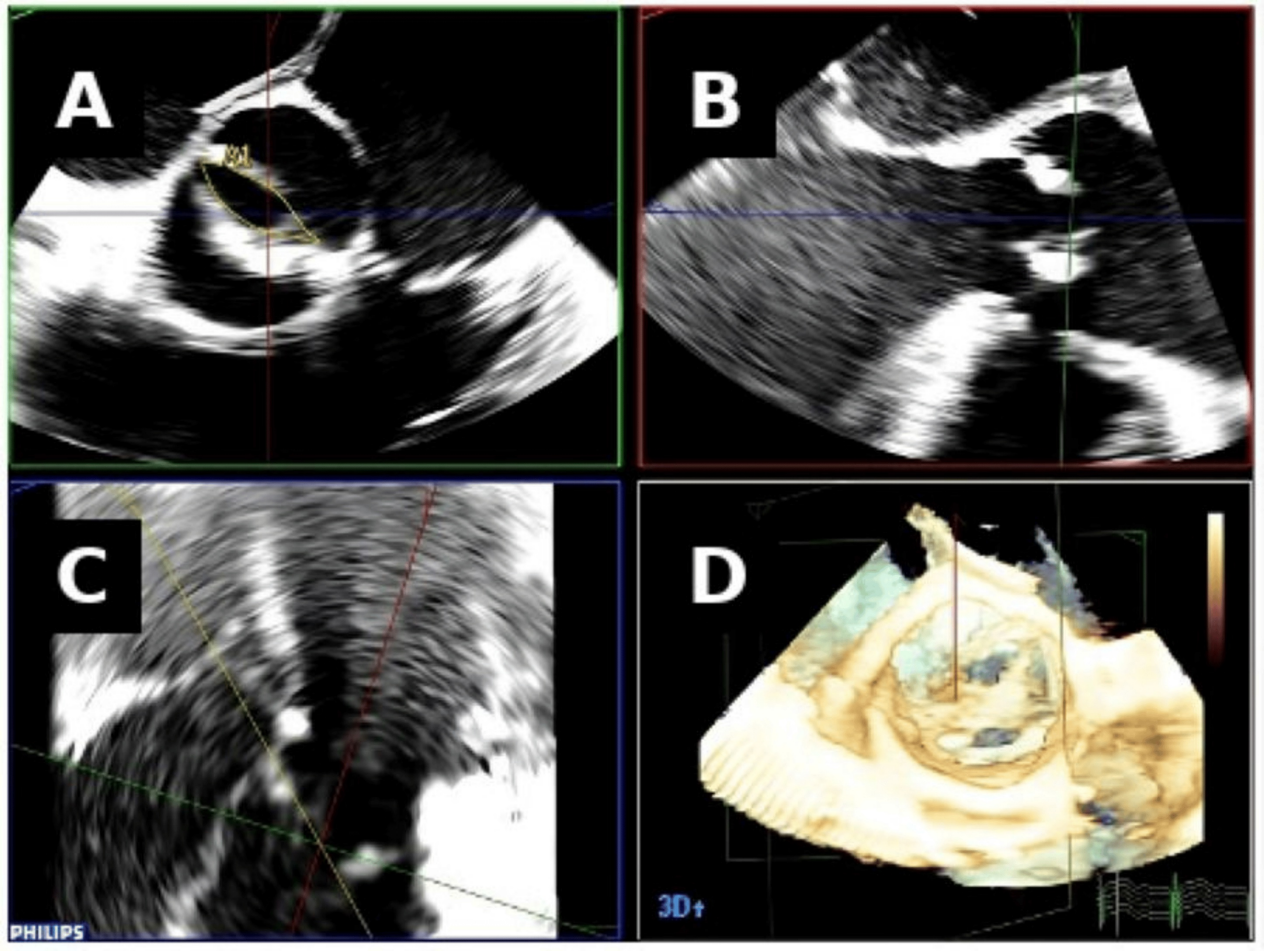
Three-dimensional transesophageal echocardiography using multiplanar reconstruction for intra-procedural assessment of bicuspid aortic valve morphology. Figure reproduced from the study by De Agustin et al. licensed under CC-BY [24].
